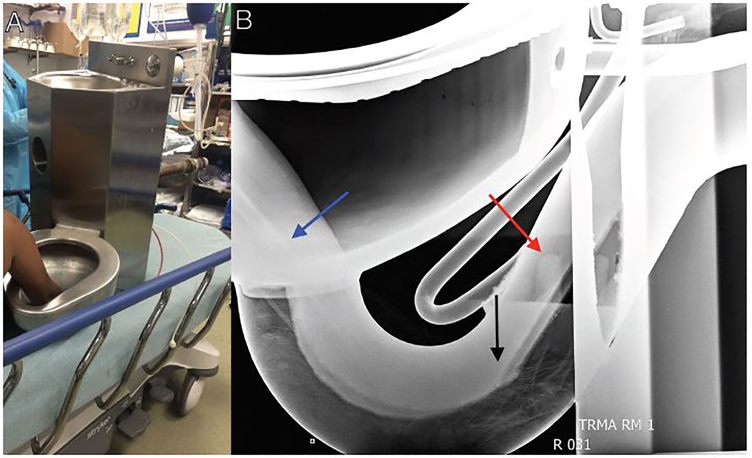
The patient’s right hand and intact sink/toilet assembly (Panel A) on the stretcher in the trauma bay; the lateral view radiograph of the entrapped hand (blue arrow – shaft of radius; black arrow – metacarpals; red arrow – distal tips of fingers) inside the toilet (Panel B).
